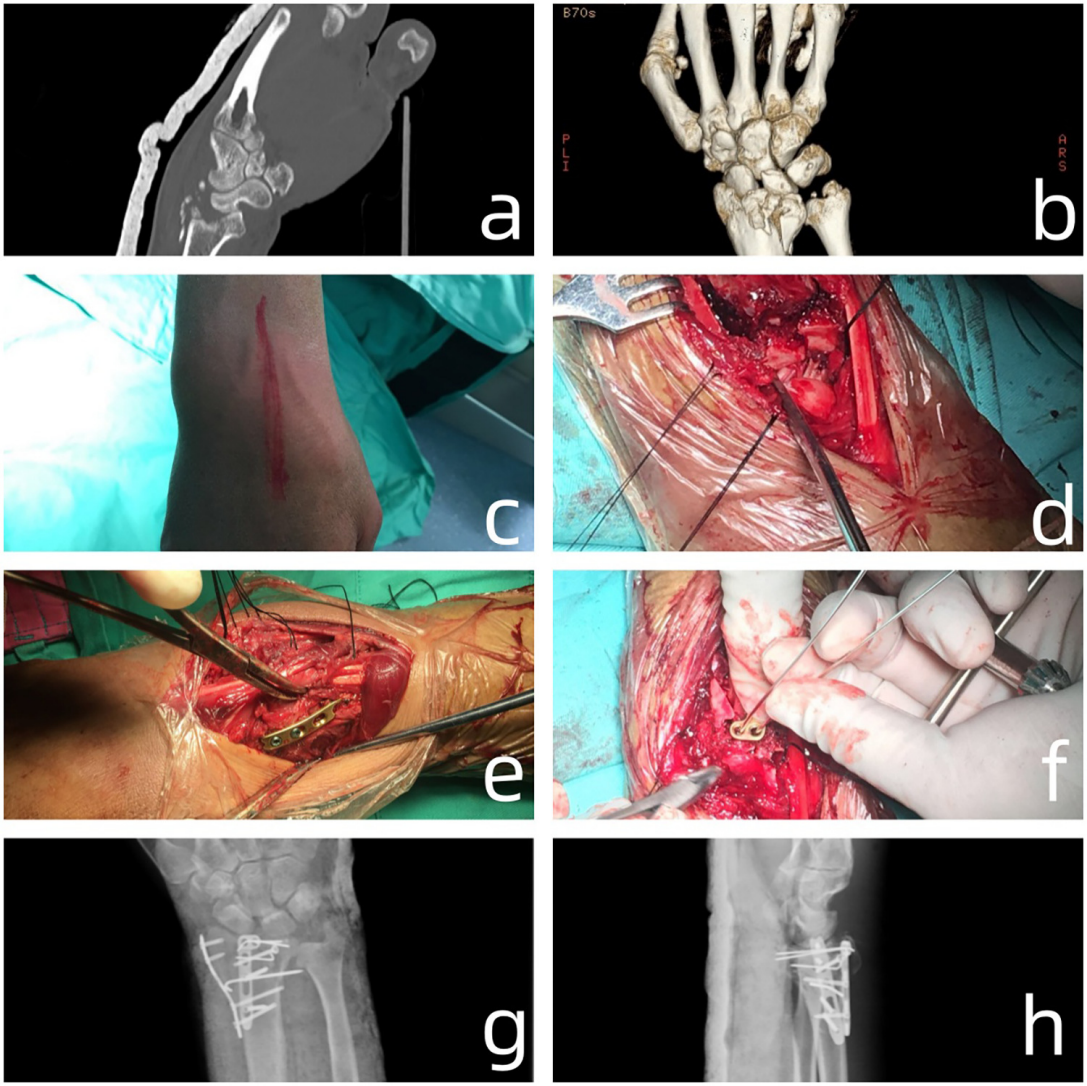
(a,b) Preoperative CT scan and three-dimensional reconstruction of the distal radius fracture showed that the dorsal articular surface collapsed; (c,d) an incision was made on the dorsal midline of the wrist, measuring approximately 7 cm in length. The subcutaneous tissue and deep fascia were incised, and the tendon sheath of the extensor pollicis longus muscle was cut. The muscle was then retracted, revealing the posterior aspect of the radius. A comminuted fracture of the distal extremity of the radius was visible, with fragmented joint surfaces and numerous small loose bone fragments; (e,f) A suitable L-shaped titanium plate was placed on the medial aspect of the radius, and it was fixed with several screws. A suitable radial plate was placed on the lateral aspect of the radius and fixed with several screws; (g,h) Postoperative radiographs of the lateral and posteroanterior views of the patient.
